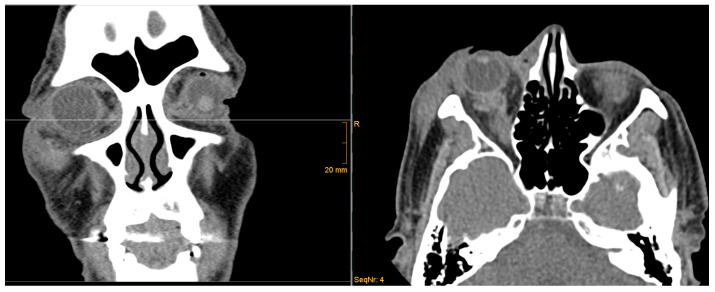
CT scan. Hyperdensity in the right retrobulbar space, swelling of periorbital tissue as evidence of RBH.
